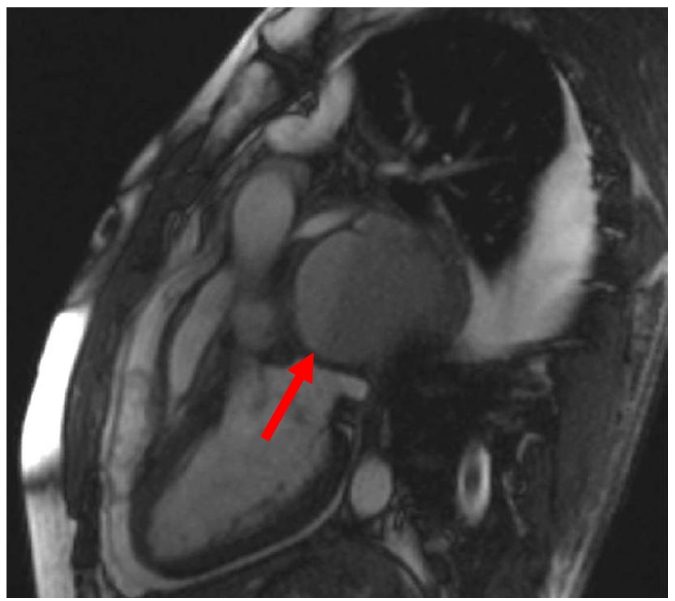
Cardiac MRI with large cyst (arrow - internal fluid level)
